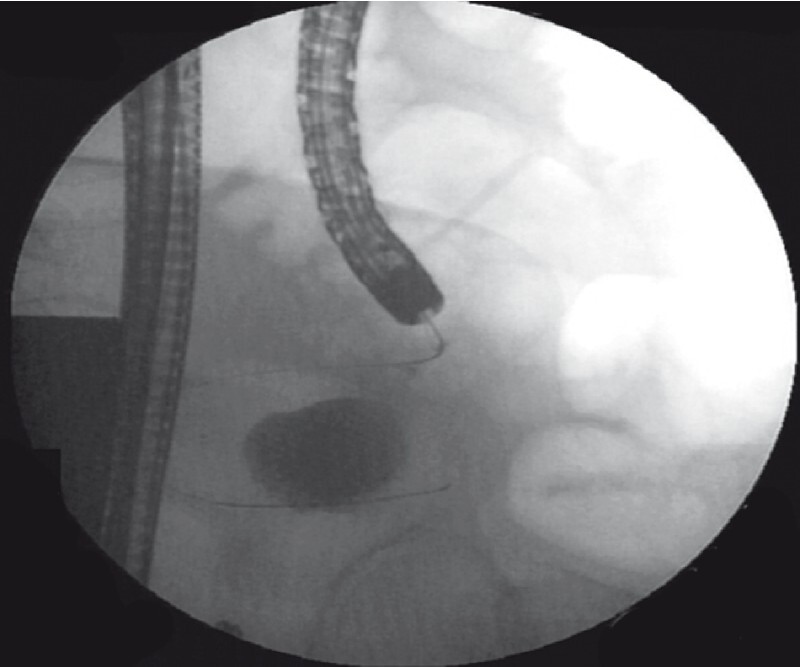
The guidewire was delivered to the appendix pus cavity, and then iohexol radiography clearly showed the giant periappendiceal abscess.
